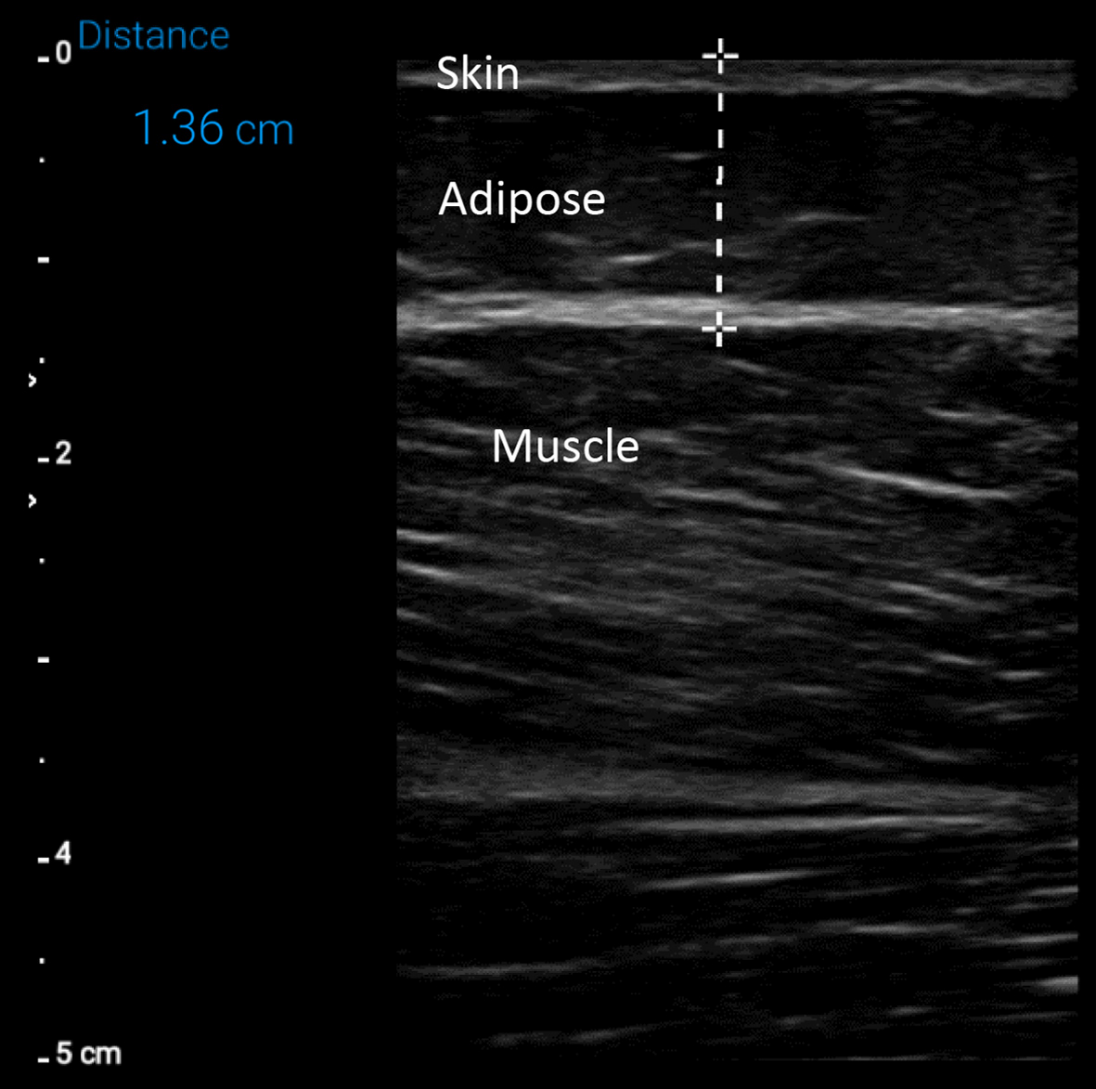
A sample ultrasound image that was used to measure SATT. In each ultrasound image, the outermost layer was skin, followed by an intermediate subcutaneous adipose tissue layer, followed by the muscle layer. SATT was determined as the distance between the outermost surface of the skin and the outermost surface of the muscle.
